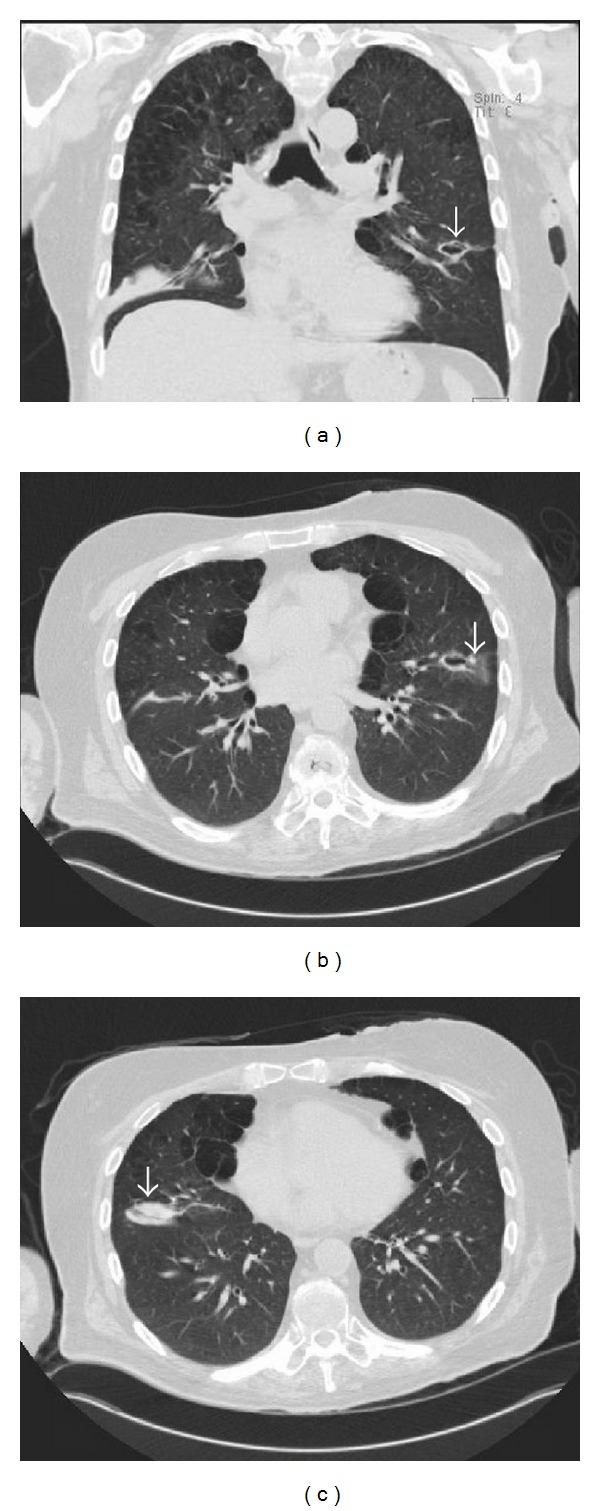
Chest CT scan. Coronal view (a) and axial views ((b) and (c)) showing a right lower lobe mass-like dense opacity with a left lower lobe cavitary lesion (arrows) and upper lobe predominant paraseptal emphysema.
